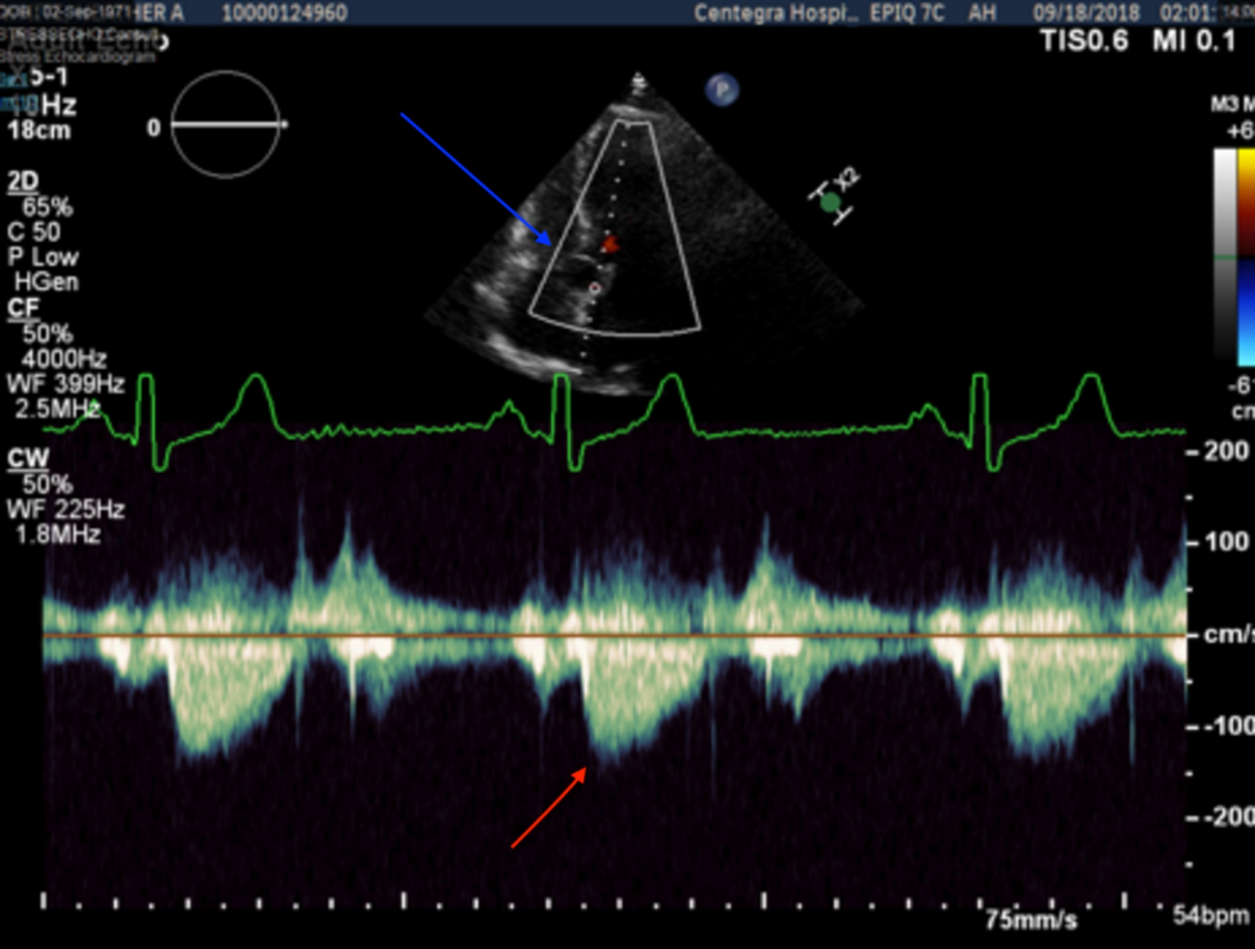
Stress echocardiography aortic view showing mitral cyst (blue arrow) and normal peak velocity with no left ventricle outflow tract obstruction pre-exercise (red arrow)
